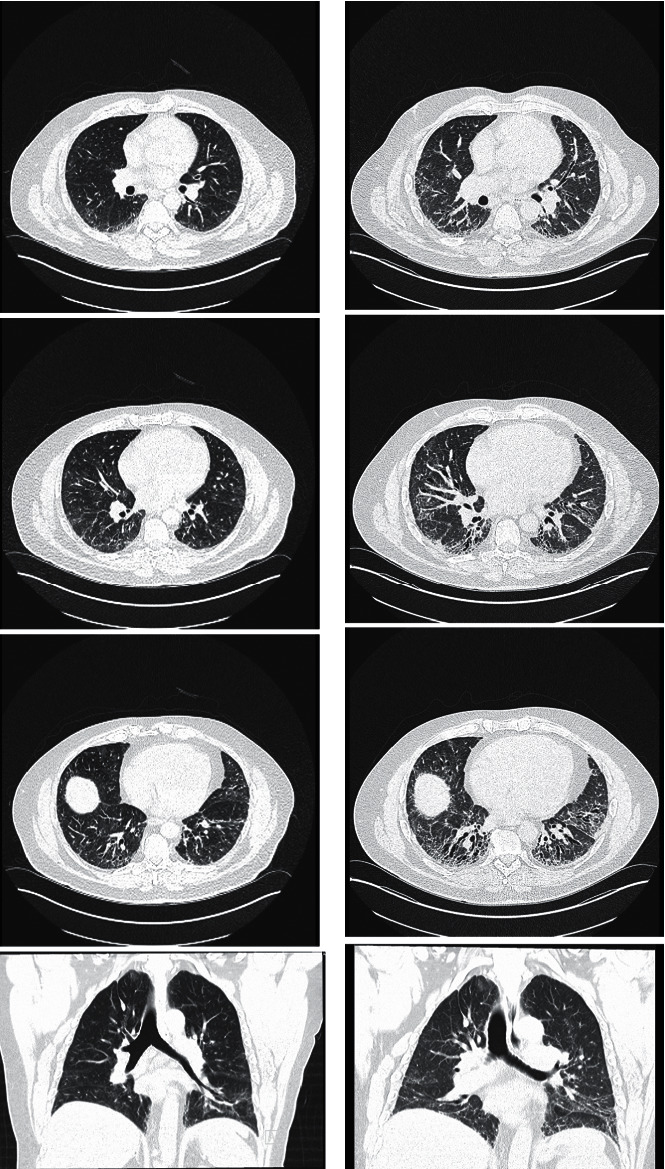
High-resolution computed tomography of the chest (Case 2). The scan 10 months prior to our evaluation (a) demonstrated a typical appearance of non-specific interstitial pneumonia (NSIP) with increased reticulation, traction bronchiectasis, lobar volume loss, and ground glass opacification in the lower lung zones. Bibasilar ground glass opacities that spare the subpleural region are characteristic for NSIP. The scan taken at our center 10 months later (b) demonstrated significant progression in fibrotic abnormalities.
